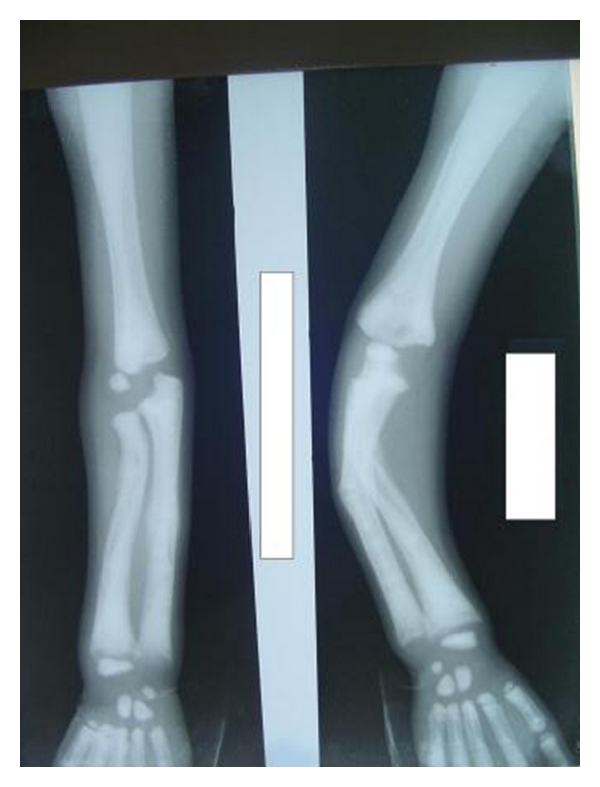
Radiograph of the upper limb showing bowing of the radius and ulnar.
